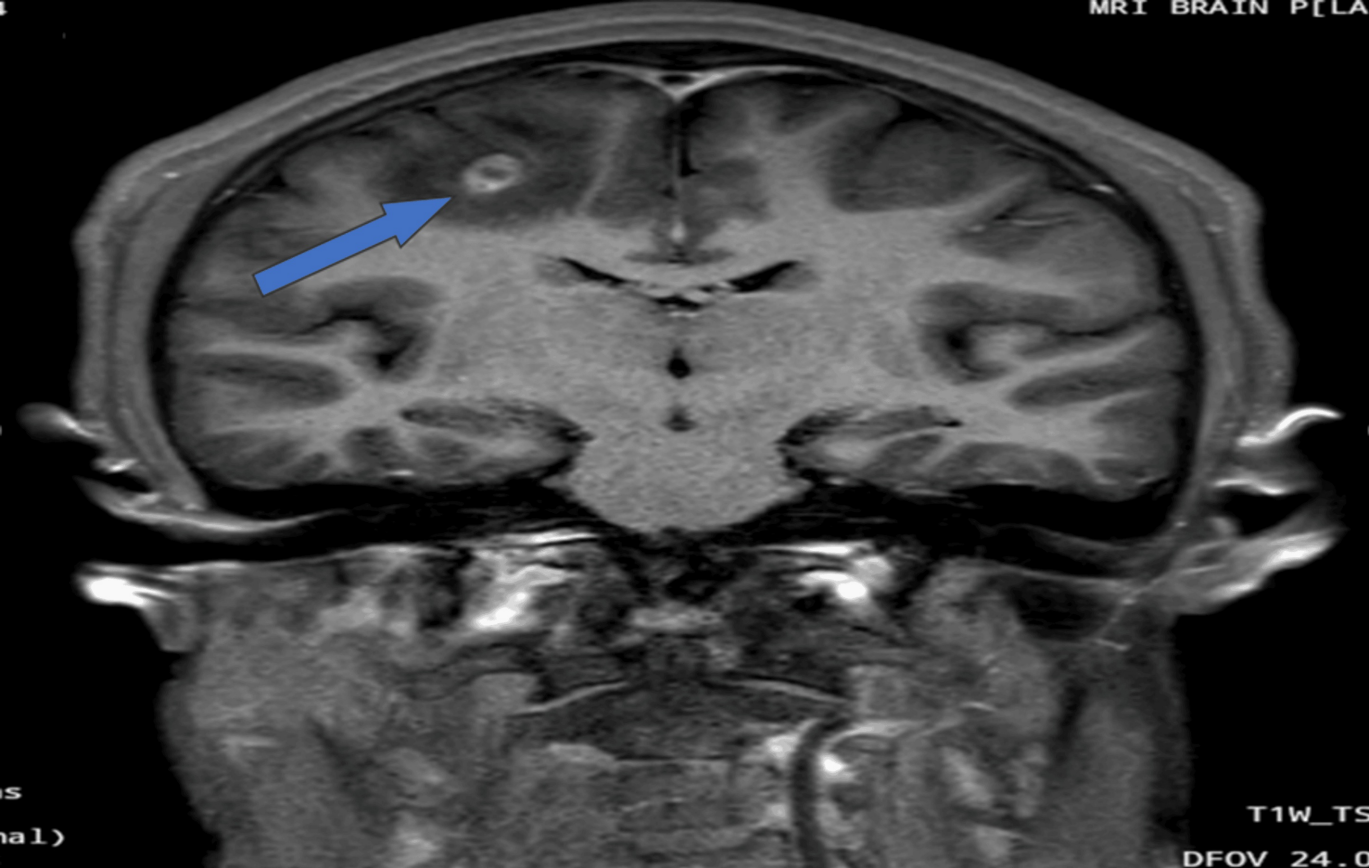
Post-contrast coronal T1-weighted MRI image showing an intensely ring-enhancing lesion in the right high frontal lobe at the gray-white matter junction, with surrounding non-enhancing hypointense perilesional edema (arrow).
